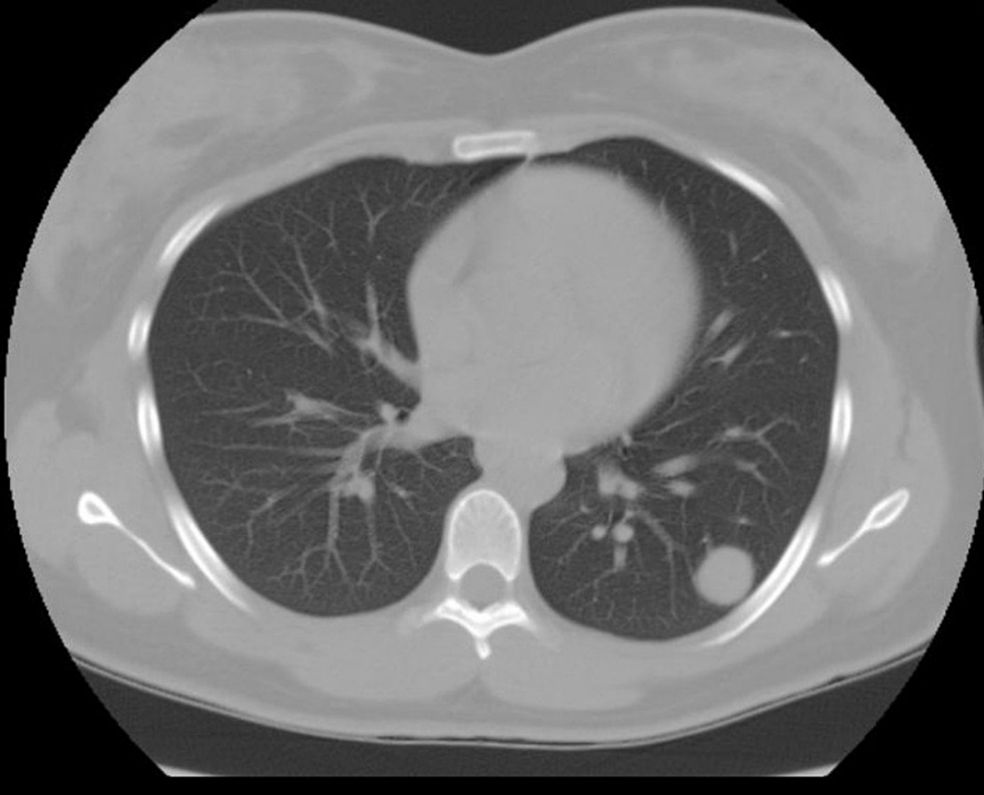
Computed tomography of chest showing pulmonary nodule. Even with the growth of nodule from its first diagnosis, it retains the non-cavitary and non-calcified features. The peripheral location of the nodule in the basilar segment of the left lower pulmonary lobe is appreciated.
